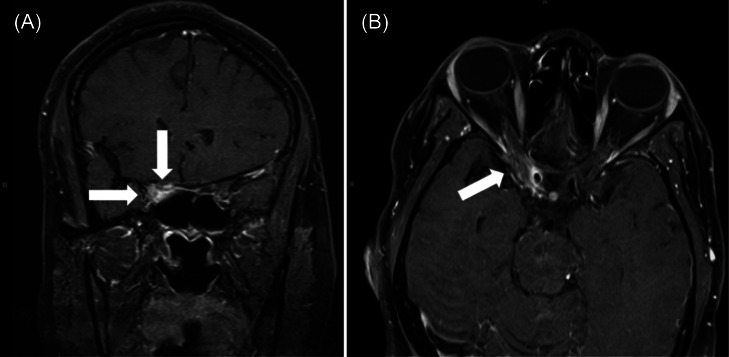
Coronal (A) and axial (B) slices of magnetic resonance imaging orbits with contrast demonstrating an abnormal signal surrounding the right cavernous sinus and petrous apex suspicious for Tolosa-Hunt syndrome (arrows).
